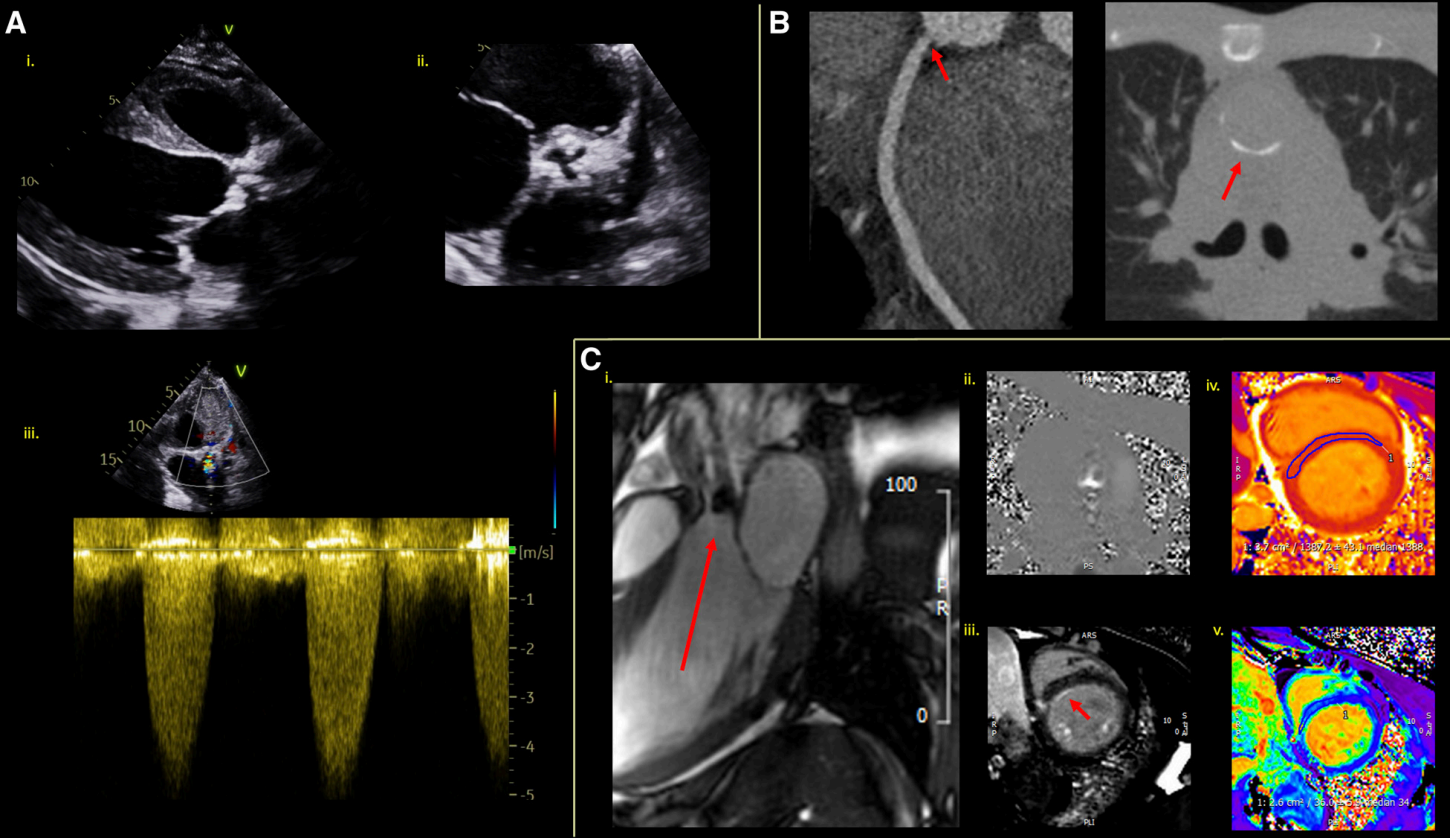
A 29-year-old man who had undergone mantle field radiotherapy for lymphoma aged 4 years. A, Transthoracic echocardiography image showing visually dilated left ventricle (i), heavy calcification of the aortic valve (ii), and severe aortic stenosis (Velocity-max of 4.57 m/s; iii). B, Cardiac computed tomography coronary angiography showing noncalcified ostial disease (left) as well as calcification of posterior aorta (right). C, Cardiovascular magnetic resonance imaging on 3T scanner showing marked aortic thickening and stenosis on 3-chamber cine (i) and flow mapping (ii), with tissue characterization showing areas of basal subendocardial and intramyocardial septal late gadolinium enhancement (iii, arrow), as well as raised septal T1 MOLLI at 1387 (normal values, 1220–1360) (iv) and raised septal extracellular volume (ECV) at 34% (v).
